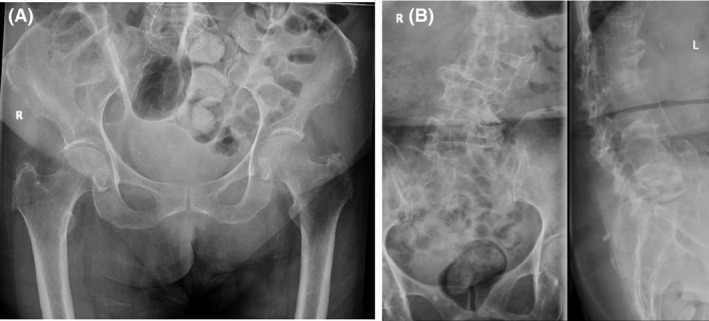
Examples of excluded radiographs (A) Gross overlying bowel gas (B) Severe scoliosis. An exclusion criteria were used to exclude radiographs such as these from this study, with overlying artefact or pathology obscuring visualisation of landmarks used for VGA scoring to prevent these negatively influencing VGA scores.
